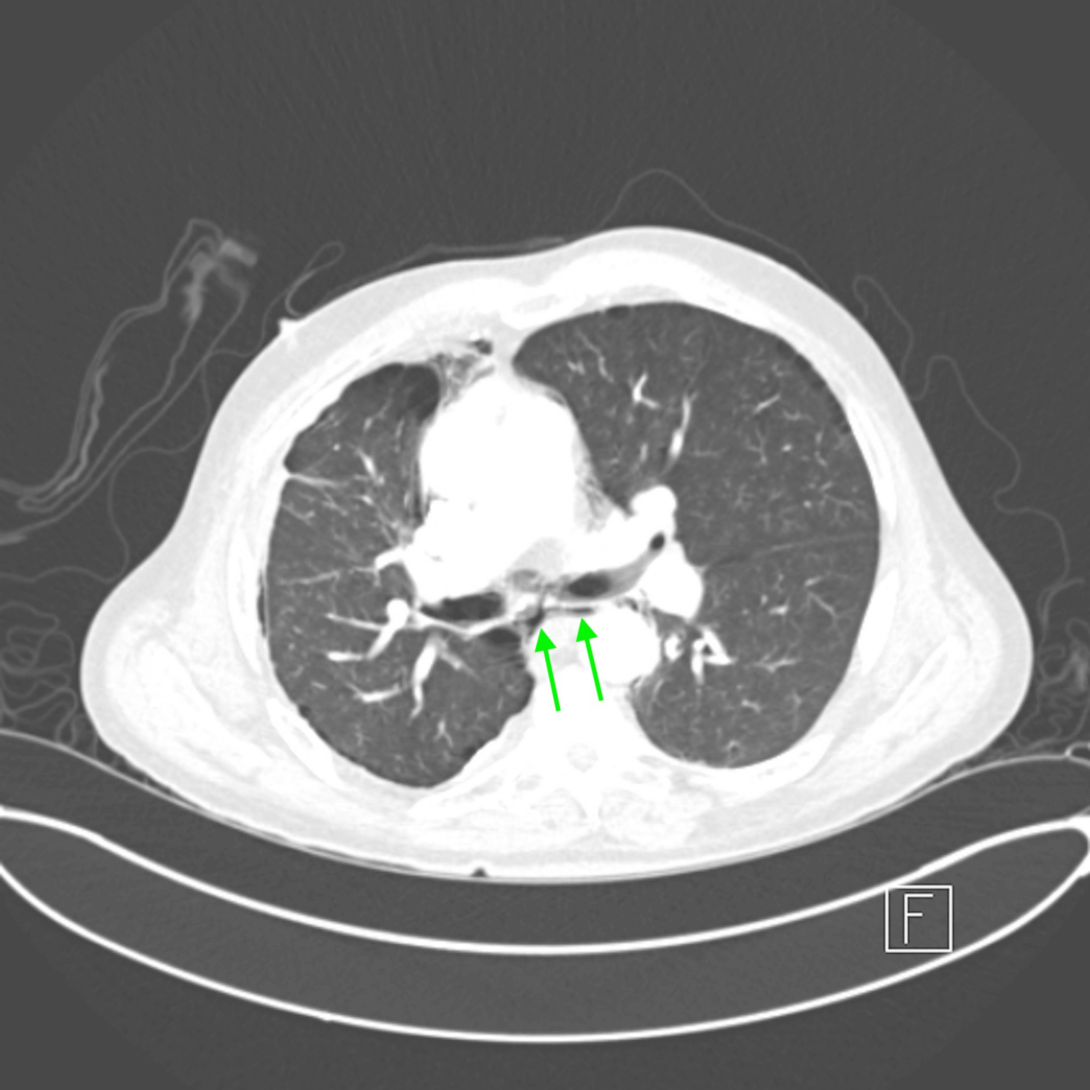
Axial view of CT angiography showing pneumopericardium Green arrows indicate pneumopericardium CT: computed tomography
